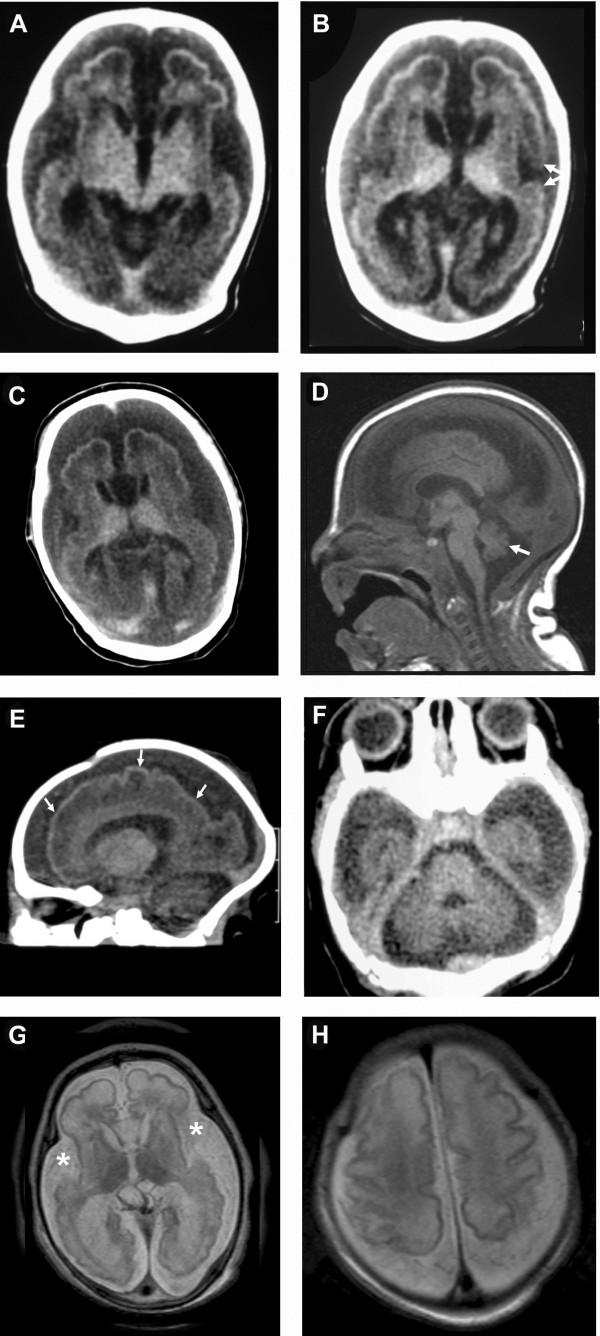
Patient 1 (Figure1, IV.1) CT axial images of the brain (A&B, age = 21 days) demonstrating brain underdevelopment with large extracerebral CSF spaces. The frontal and temporal lobes sulci [A] are more developed compared to Patient 6 [G&H], but still immature. The Sylvian fissures [B] are more developed and have visible frontal and temporal opercula (arrows). [C&D]. Patient 2 (Figure
